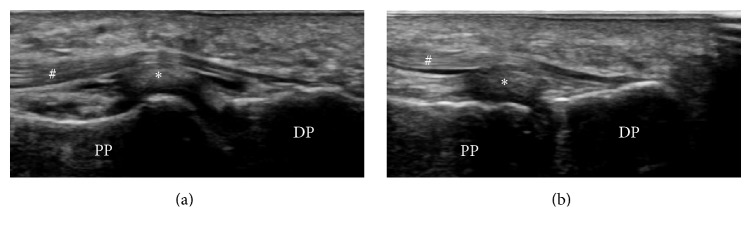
Ultrasonography image of the interphalangeal joint (IPJ) showed fluid retention around the plantar capsule nodule and flexor hallucis longus tendon on the affected side, but not on the healthy side, of the great toe. (a) Affected side. (b) Healthy side. DP: distal phalanx and PP: proximal phalanx. ^*∗*^Plantar capsule nodule. ^#^Flexor hallucis longus.
